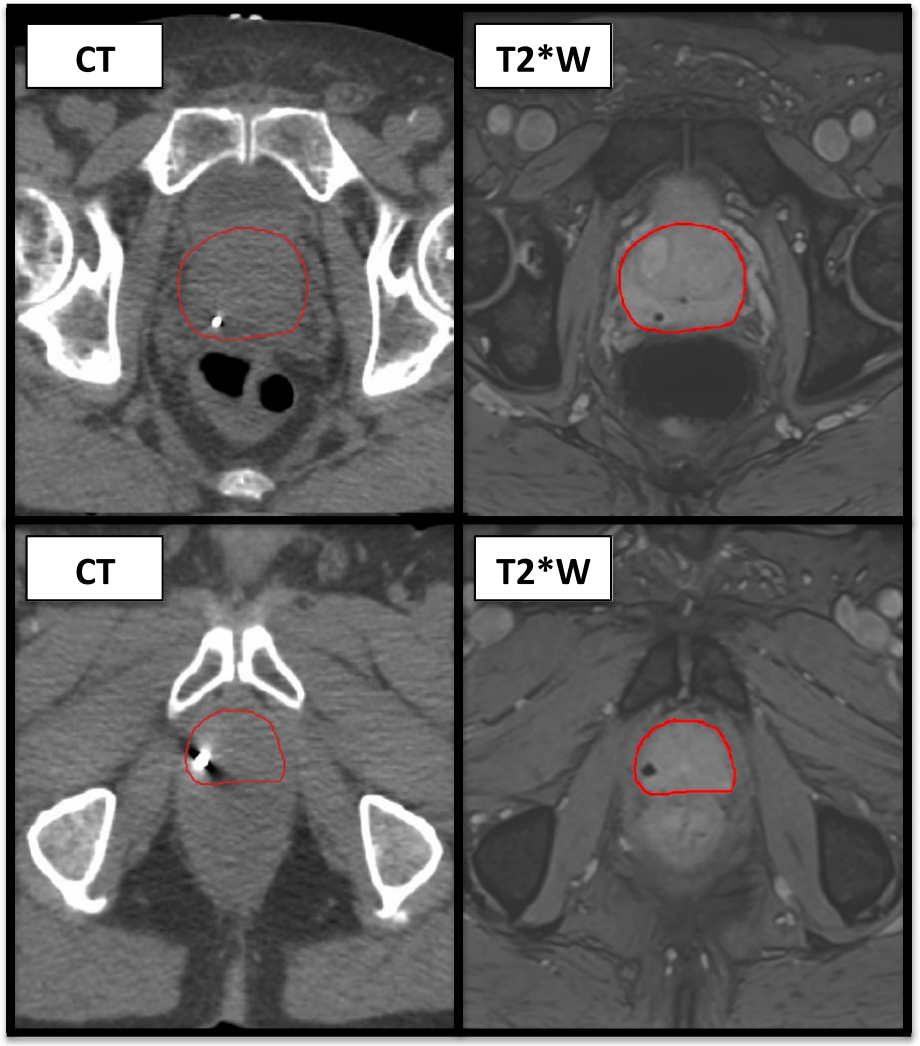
Clinical example of the variation in signal loss. Top line‐CT (left) and T2*W (right) imaging displaying the usual signal loss associated with a fiducial marker in the cranio‐caudal position. Bottom line‐CT (left) and T2*W (right) imaging for the same patient showing the altered signal loss seen with the inferior fiducial marker which in this case is angled more in the transverse plane.
